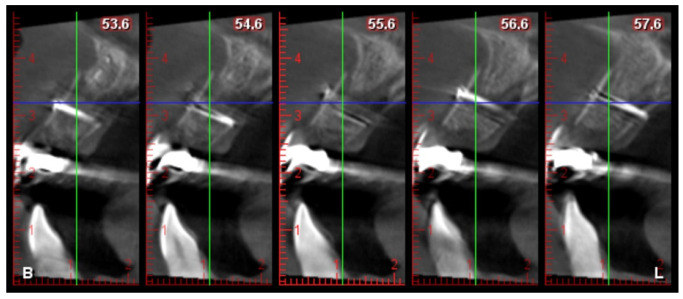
Post-operative CBCT cross sections of the augmented site.
